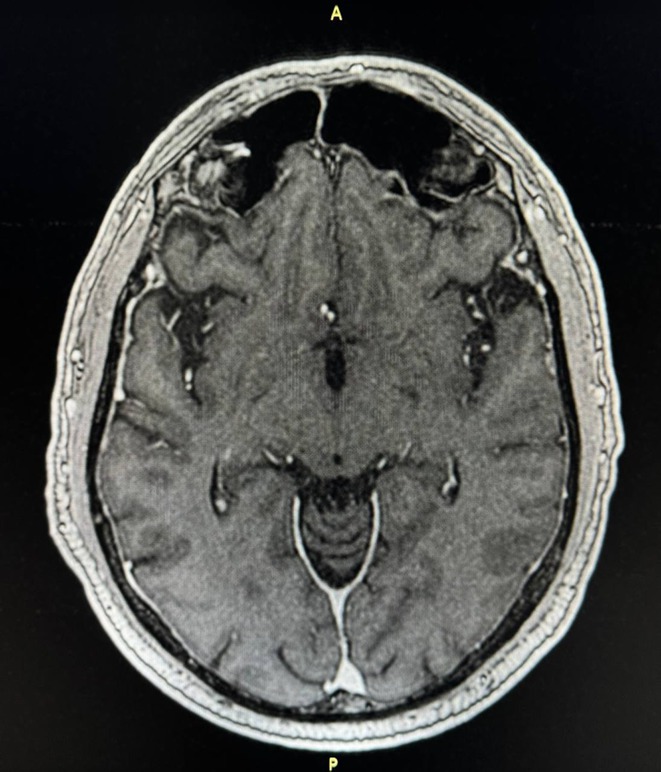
FIGURE 2 Patient's brain MRI T1 post contract sequence, axial projection ‐leptomeningeal post‐contrast enhancement.
